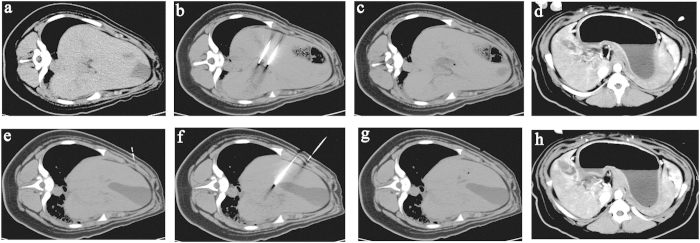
CT scan before and after treatment. The upper four CT scans are the liver before treatment (a), the two-needle electrodes inserted in the hilus hepatic (b), 2 hours after treatment (c) and 24 hours after treatment (d). The lower four CT scans are the gall bladder before treatment (e), the two-needle electrodes inserted near the neck of the gall bladder (f), 2 hours after treatment (g) and 24 hours after treatment (h). The CT images demonstrated a clear hypoattenuating area with a hyperattenuating rim in the ablated area.
